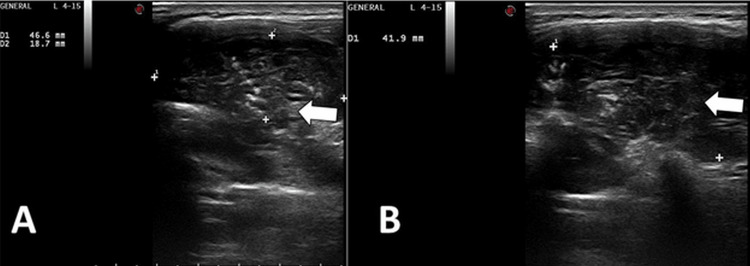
Ultrasound scan of the lump Axial (A) and sagittal (B) view shows a heterogeneously hypoechoic lesion (arrow) involving soft tissue and left paravertebral muscles with deeper extension into posterior intervertebral space.
